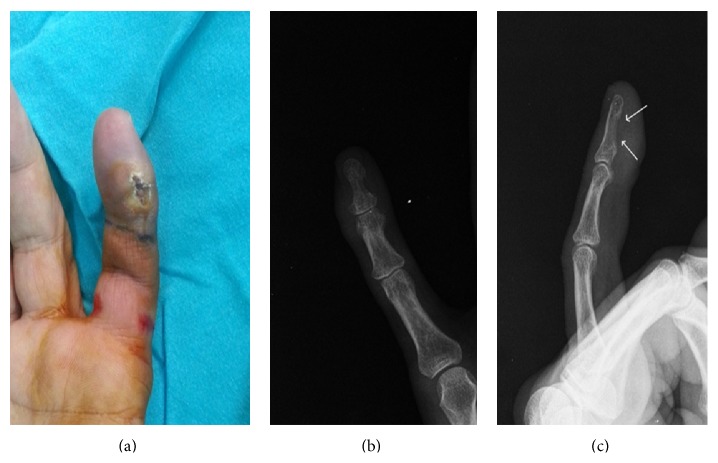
(a) Painful ulcerated lesion on the finger pulp on the left hand's small finger. ((b), (c)) PA and lateral radiographs. (c) Arrows in cortical bone and soft tissue increased radiolucent areas.
